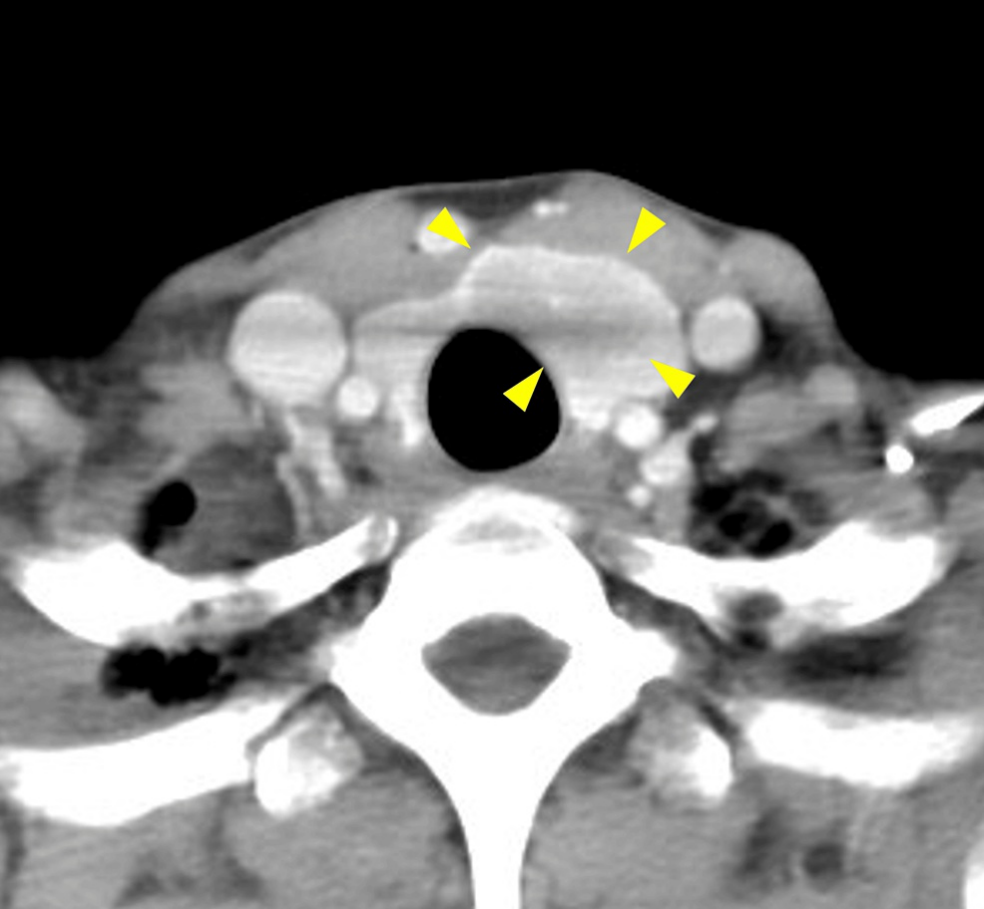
CT (axial) A low-density area from the left lobe to the isthmus CT: computed tomography
